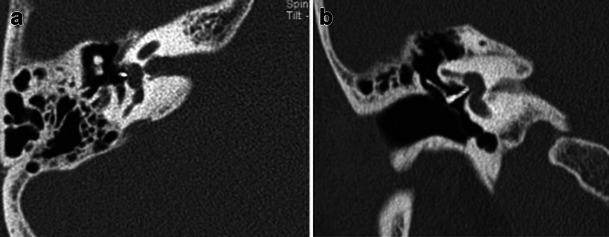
Axial (a) and coronal (b) HRCT images of the right temporal bone in a patient with stapedectomy. The radiodense stapes prosthesis is well positioned with its distal end against the oval window
